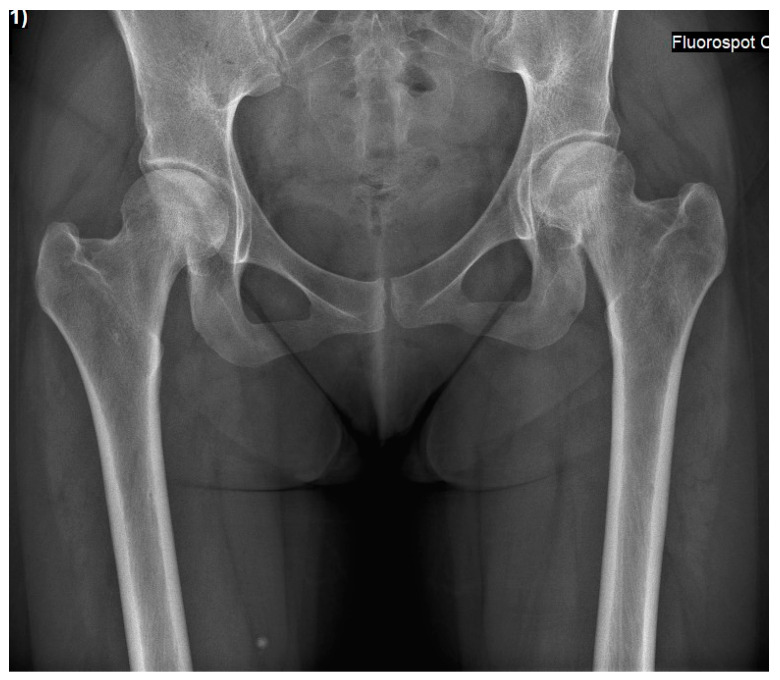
An antero-posterior pelvis X-ray of Case 2 was performed a few days after pain started, and it was already bilateral. These findings present a pre-collapse radiographic stage, where both femoral heads are spherical, and joint space is preserved.
